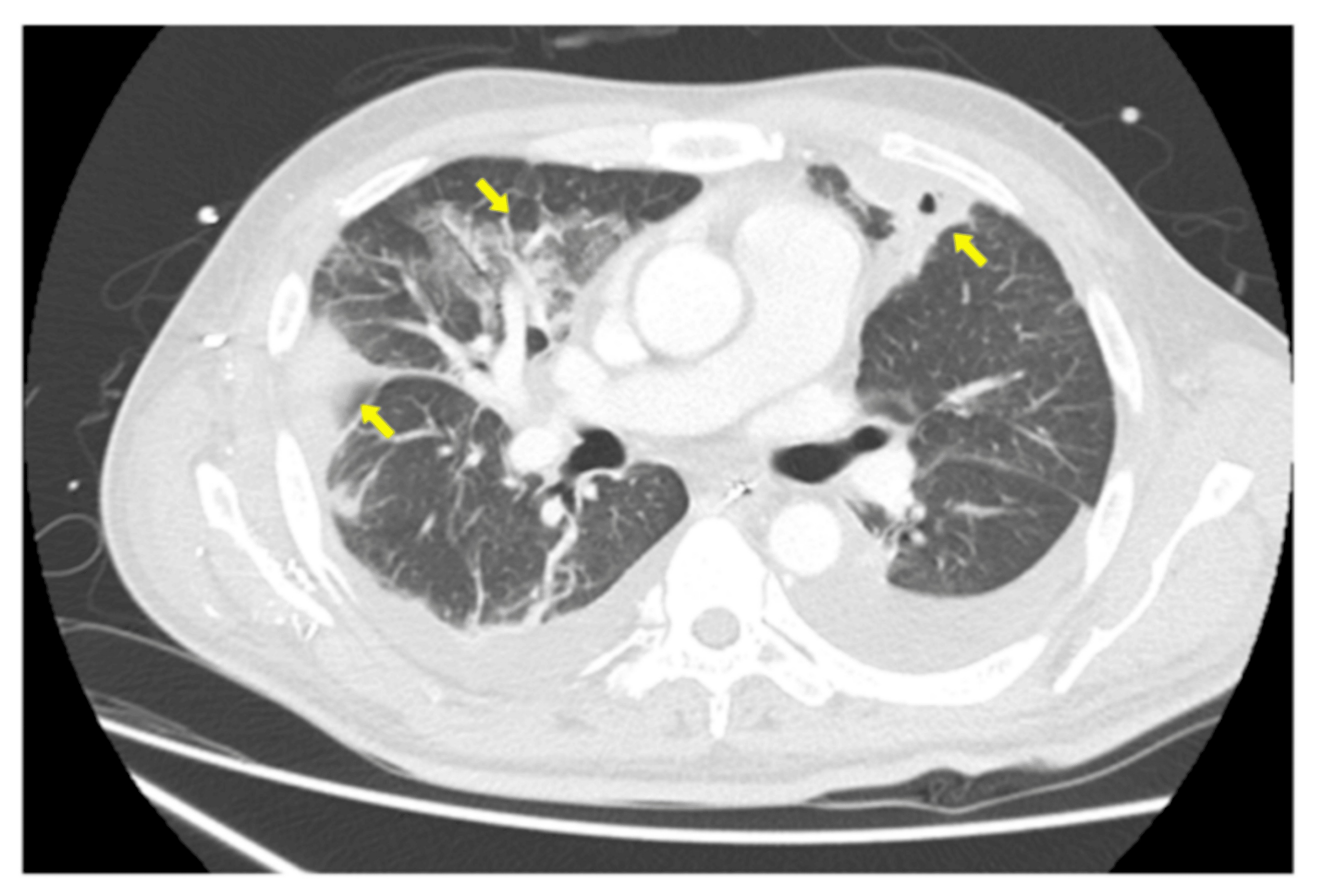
Follow-up contrast-enhanced axial computed tomography of the chest. This image demonstrates multiple lung abscesses on hospital day seven.
